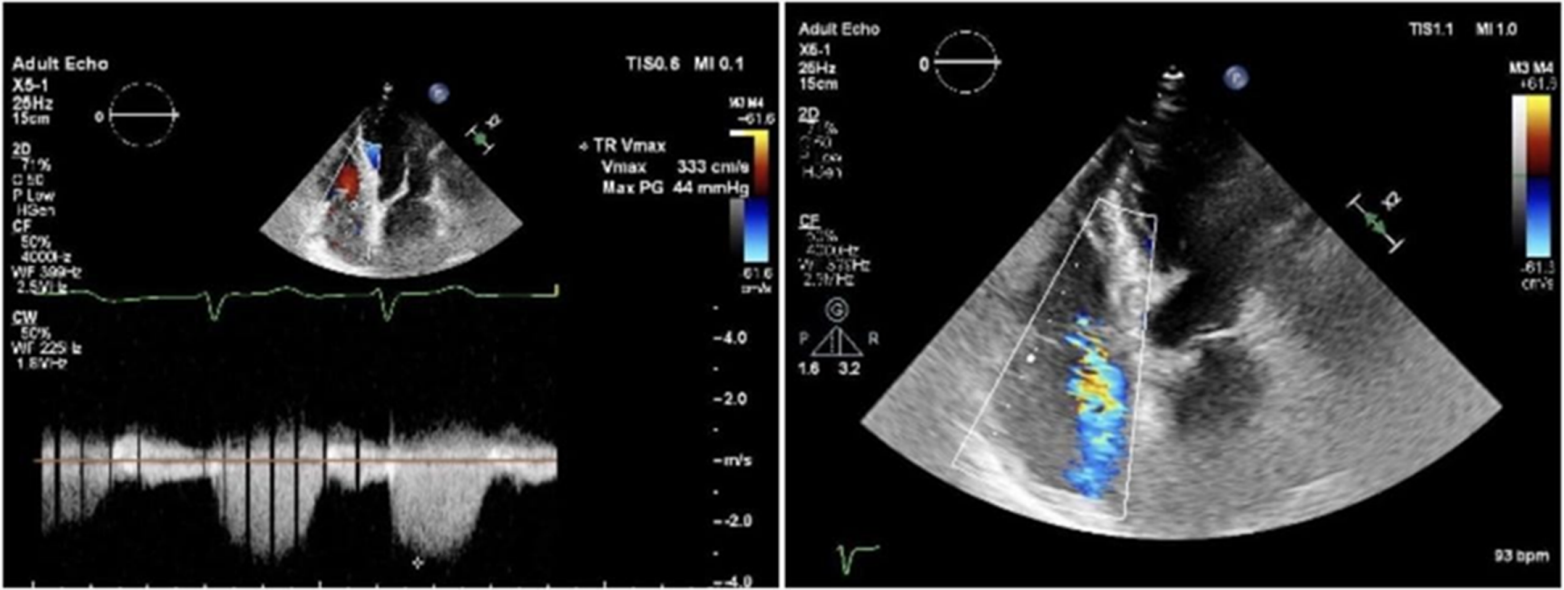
Postoperative echocardiogram showing preserved LV systolic, but dilated RV with reduced contraction and moderate tricuspid regurgitation.
